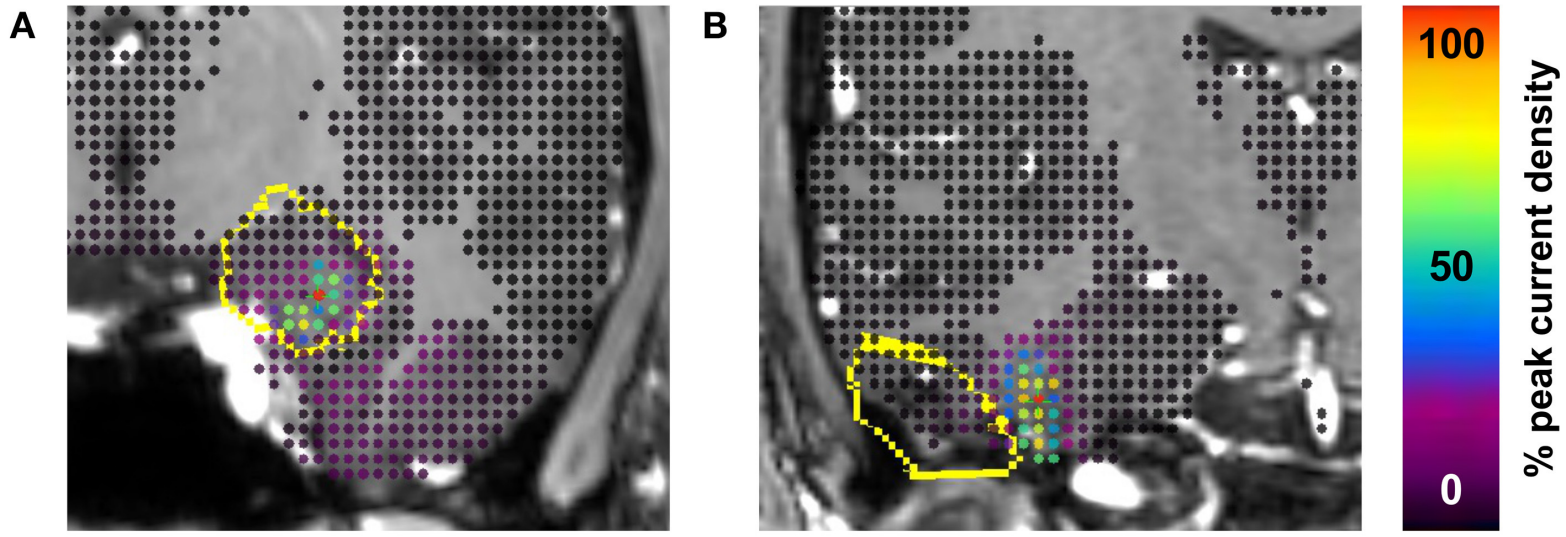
Examples of true positive and true negative cases. (A) Engel class I outcome 2 years after laser ablation of amygdala and hippocampus including 92% of interictal current. (B) Engel class III outcome 2 years after resection of temporal pilocytic astrocytoma including 0% of interictal current. The treatment volume (dilated by 2 mm to account for grid size) is outlined in yellow on coronal section from preoperative MRI. Current density is shown at each point in the gray matter grid. Proportions of current included in the treatment volume are based on the SWARM method with a lower cutoff value of 50% of the maximum point current.
